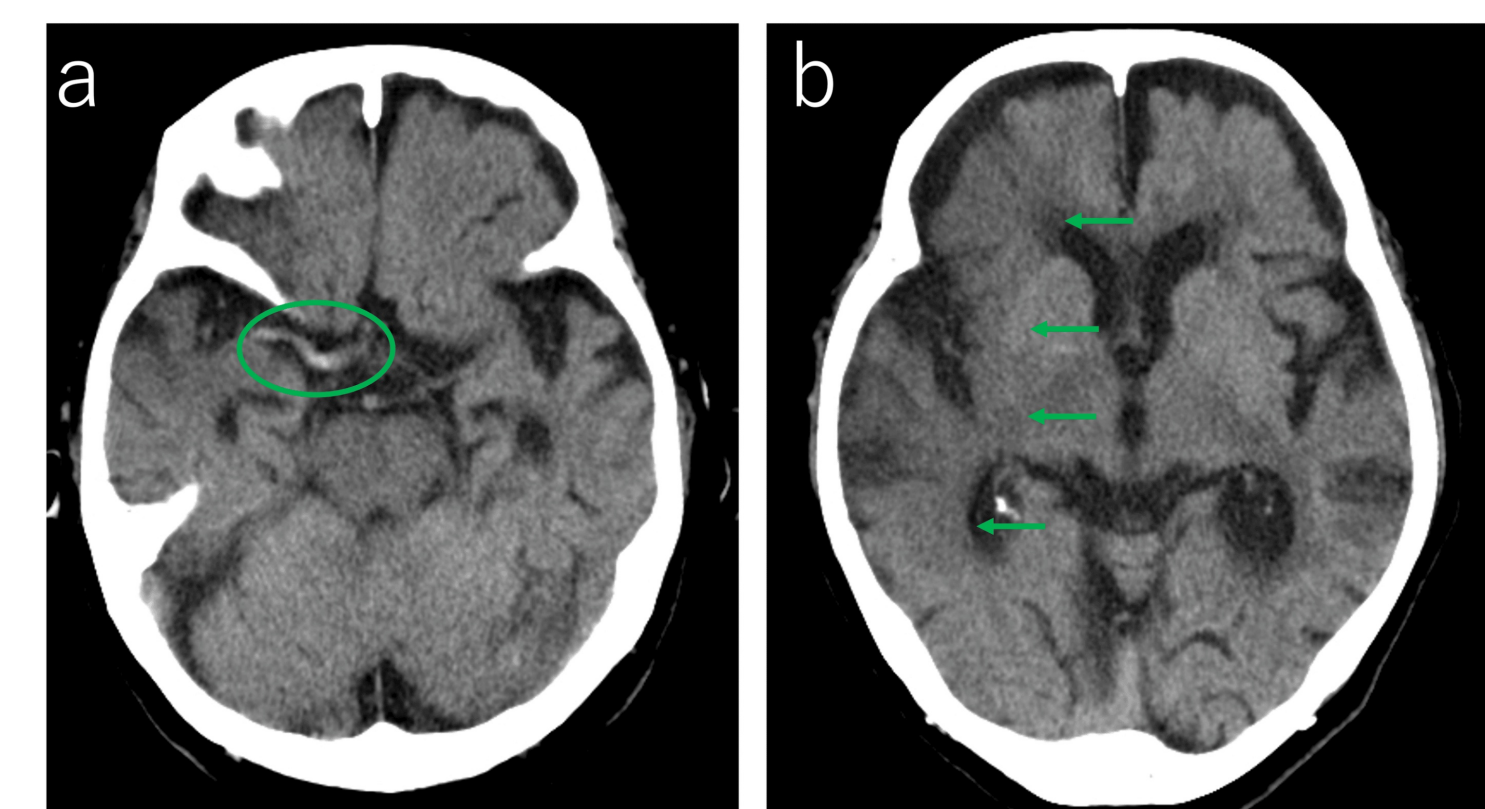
Non-contrast axial brain CT image. (a) A hyperdense sign is observed in the right middle cerebral artery (green circle); (b) loss of gray-white matter differentiation (green arrow). The Alberta Stroke Program Early CT Score (ASPECTS) was rated as 4/10 points.
